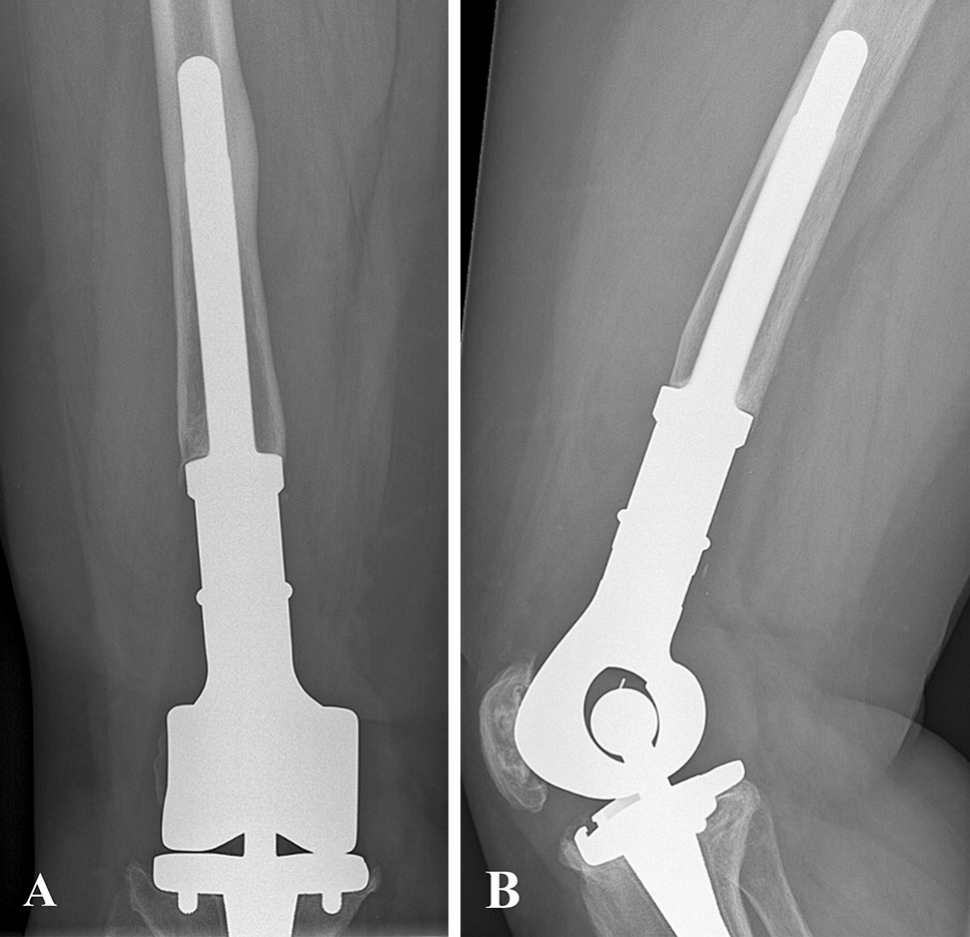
Conventional AP (A) and lateral (B) radiographs taken 6 years after extraarticular resection for an osteosarcoma of the distal femur in a 46-year-old female patient. The defect was reconstructed with an uncemented HA-coated MUTARS^®^ distal femoral replacement with a PEEK-OPTIMA^®^ locking mechanism. The postoperative course was uncomplicated and no further procedures were undertaken.
